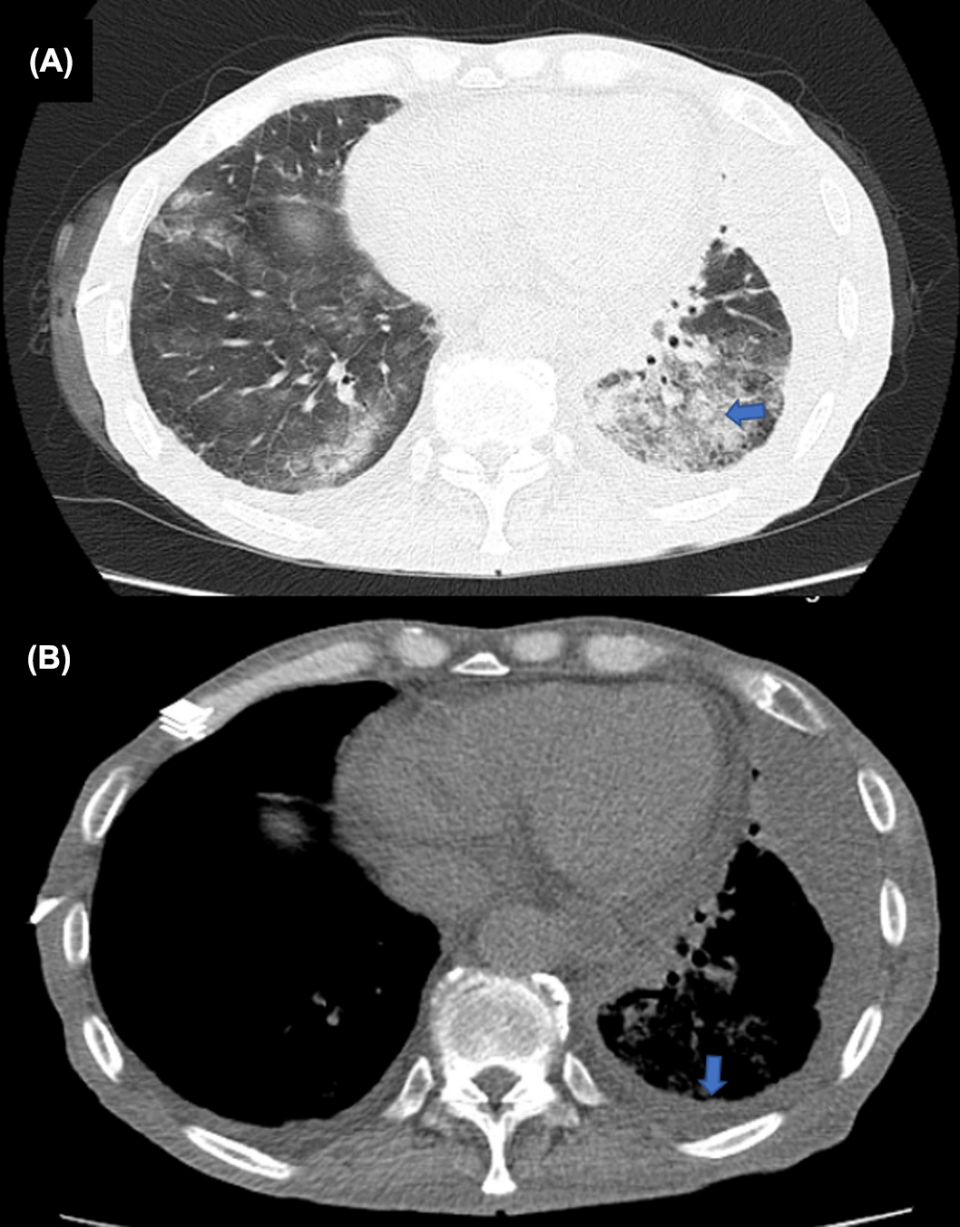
It depicts chest CT without contrast, (A) demonstrates lung window, sub pleural patchy nodular grand glass opacities of left lower lobe is seen, which is consistent with atypical pneumonia (rule out metastasis); (B) demonstrates mediastinal window, mild pleural effusion is seen.
